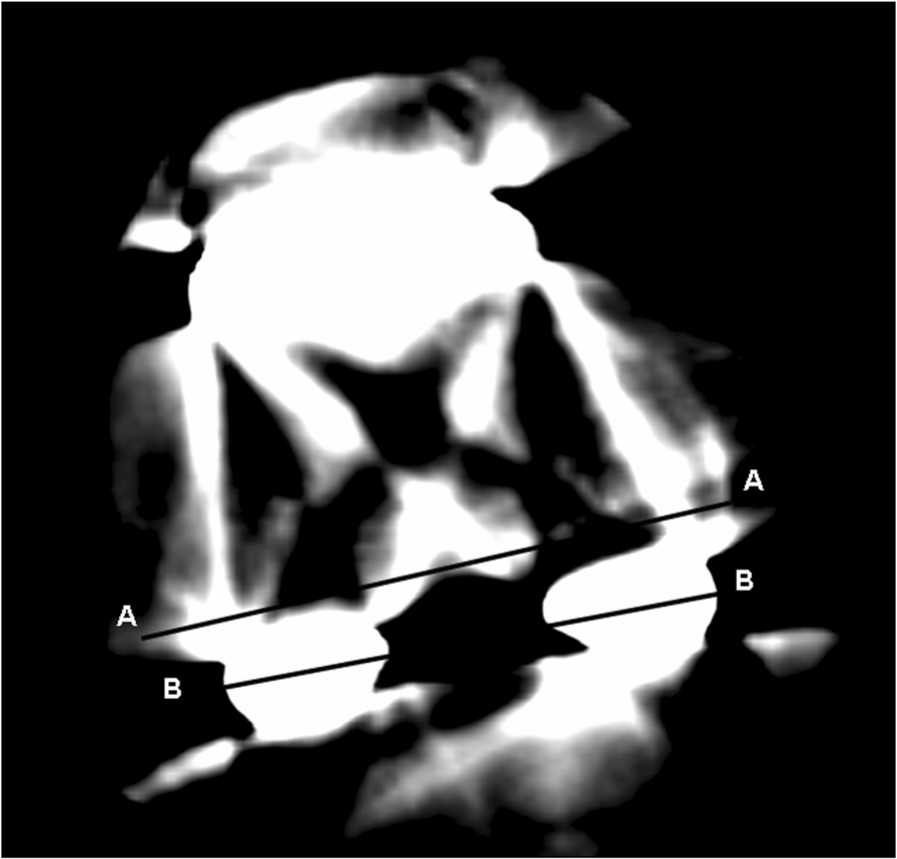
Computed tomography showing measurement of rotation of the femoral component in the axial plane. The femoral rotational (FR) angle was defined as the angle between the surgical epicondylar axis (*AA*) and the tangent to the posterior femoral condyles of the femoral component (*BB*). Measurement of computed tomography originally appeared in J Bone Joint Surg Br. 2004;86:818
